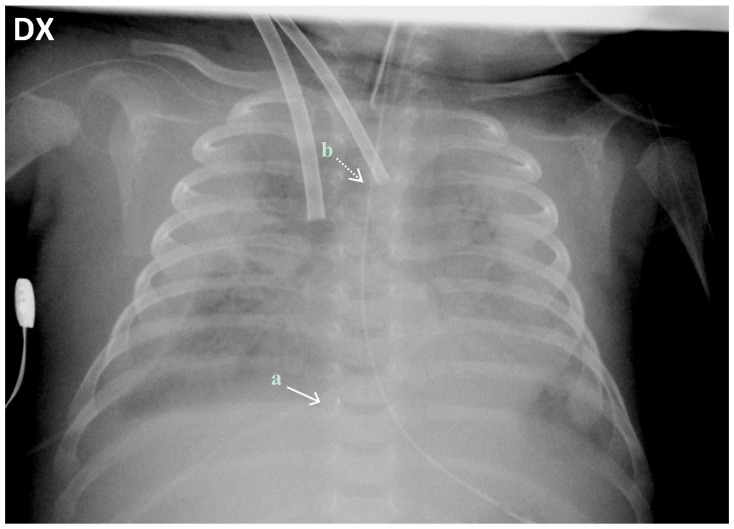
Chest X-ray of the patient 48 h after the start of ECLS support. Correct positioning of the (a) distal tip of the drainage cannula in the junction between the superior vena cava and the right atrium (straight white arrow) and (b) distal tip of the inflow cannula in the junction between the right common carotid artery and the aortic arch (dotted white arrow).
